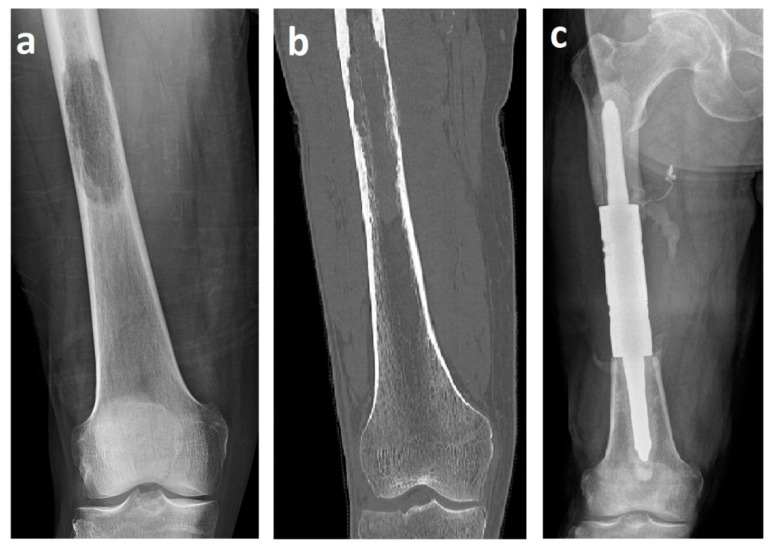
X-ray (a) and computed tomography (b) images of renal cell carcinoma solitary metastasis to the femoral diaphysis. X-ray image (c) after resection with a cemented intercalary endoprosthesis. These images are from patient 2 in Table 1.
